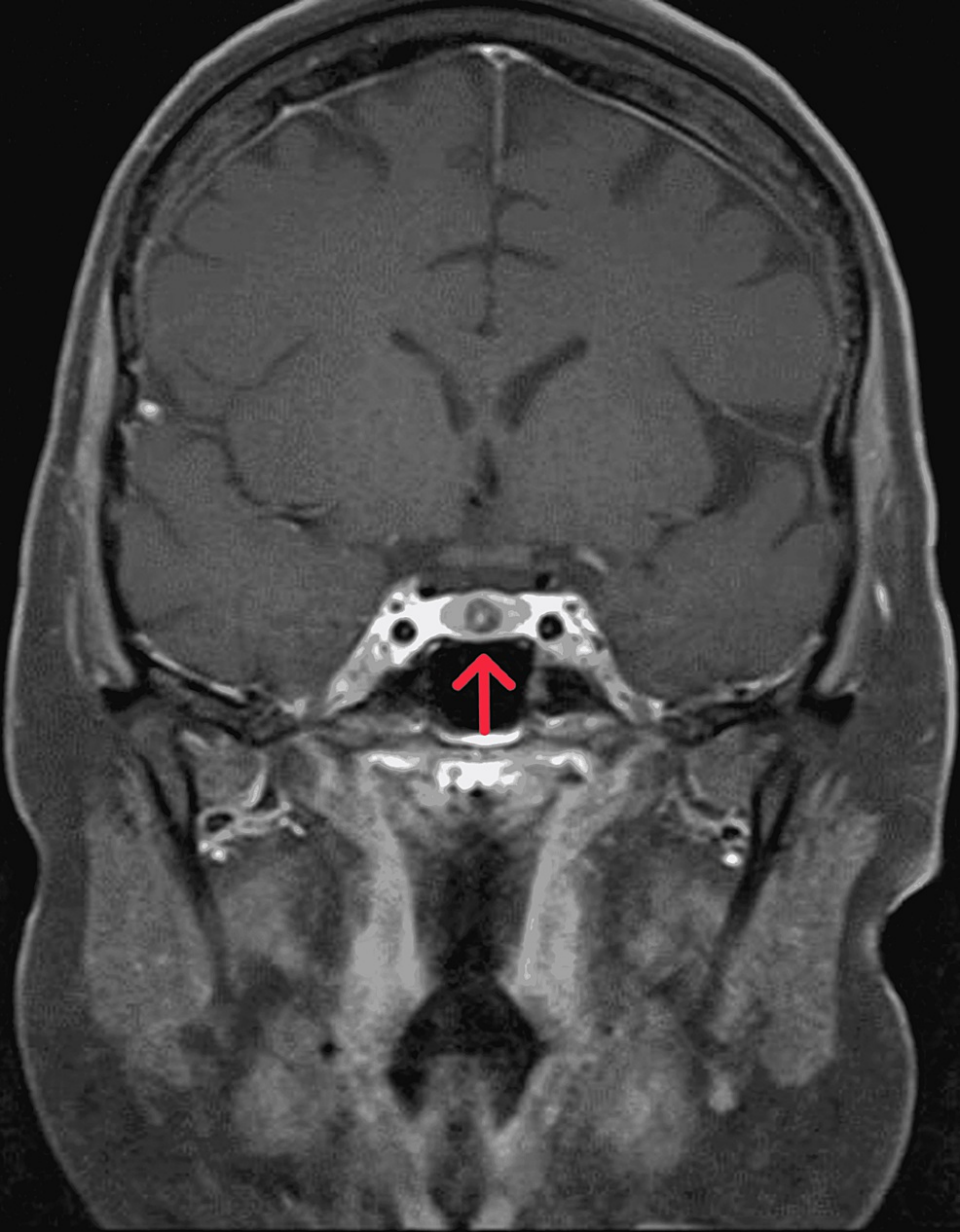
Coronal T1-weighted MRI of the pituitary gland with contrast showed a hypoenhancing nodular lesion at the midline of the anterior pituitary, with mild eccentric to the right
